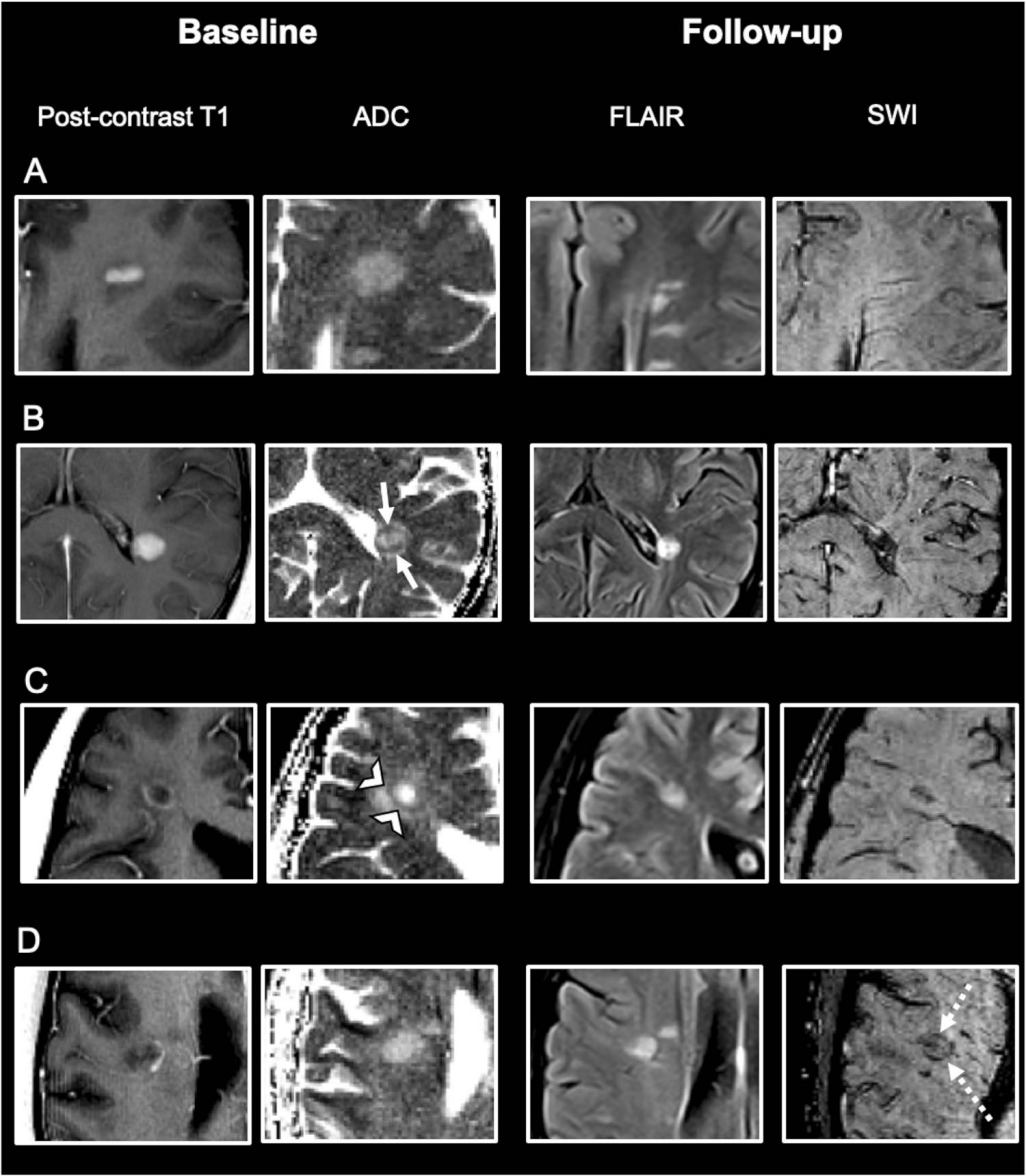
Representative examples of the observed contrast-enhancement and apparent diffusion coefficient (ADC) patterns of acute multiple sclerosis lesions. From left to right: Baseline post-contrast T1-weighted images and baseline ADC maps. Fluid-attenuated inversion recovery (FLAIR) and susceptibility-weighted images (SWI) at follow-up. A Nodular enhancement without a hypointense rim on ADC maps. On follow-up MRI after 9 months, no iron rim is detectable. B Nodular contrast-enhancement with a hypointense rim on ADC maps (arrows). On follow-up MRI after 3 months, no iron rim is detectable. C Ring-like enhancement with a hypointense rim on ADC maps (open arrowheads). On follow-up MRI after 6 months, no iron rim is detectable. D Ring-like enhancement without a hypointense rim on ADC maps. Note the iron rim on follow-up after 14 months (dotted arrows)
